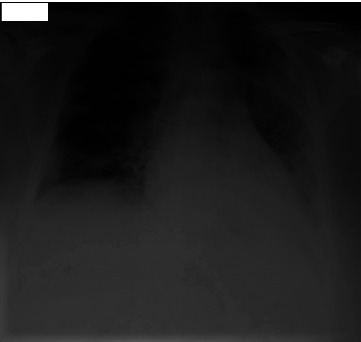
March 2020 chest X-ray. Large consolidation area at the left lung. Another consolidation area is visible at the right lung base. Large ground glass area affecting lower left lung, with minimal pleural effusion.
